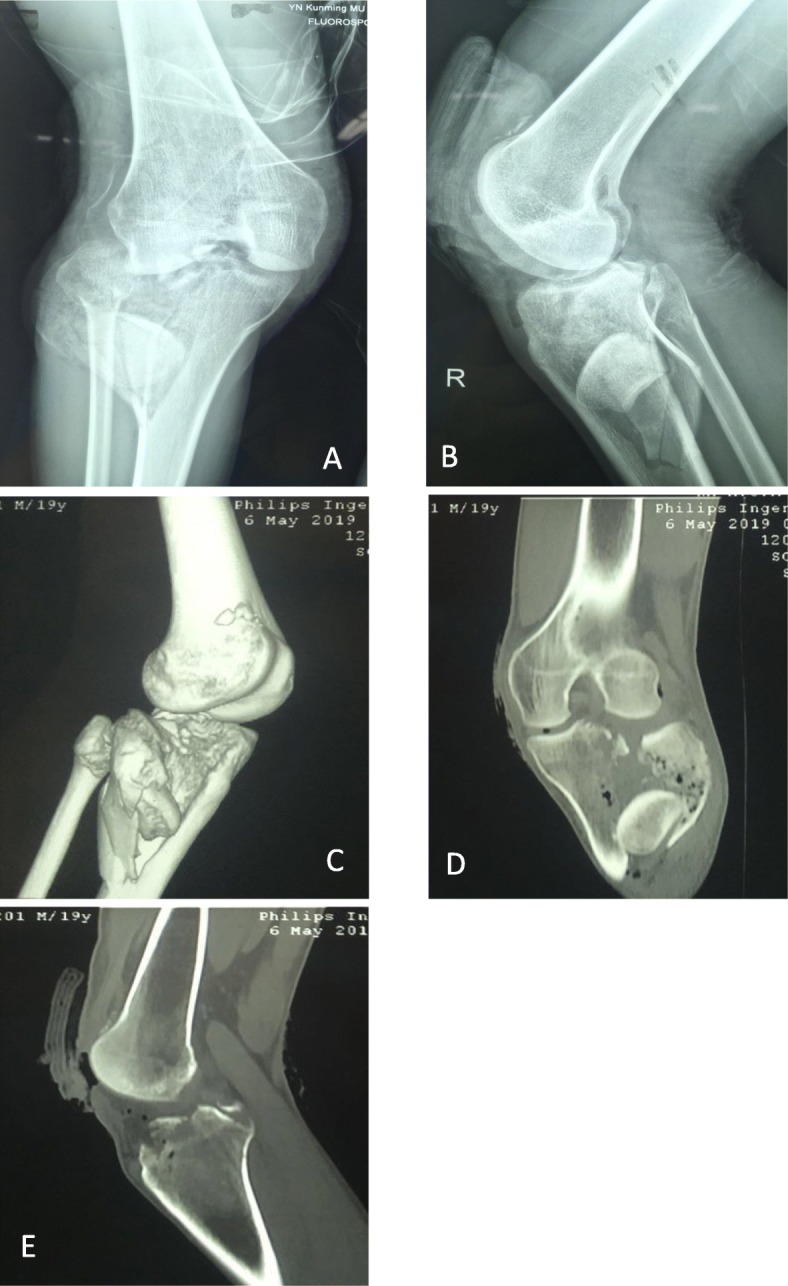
Preoperative radiographs: a AP view of radiograph; b lateral view of radiograph; c 3D CT scan; d coronal view of CT scan; e sagittal view of CT scan
